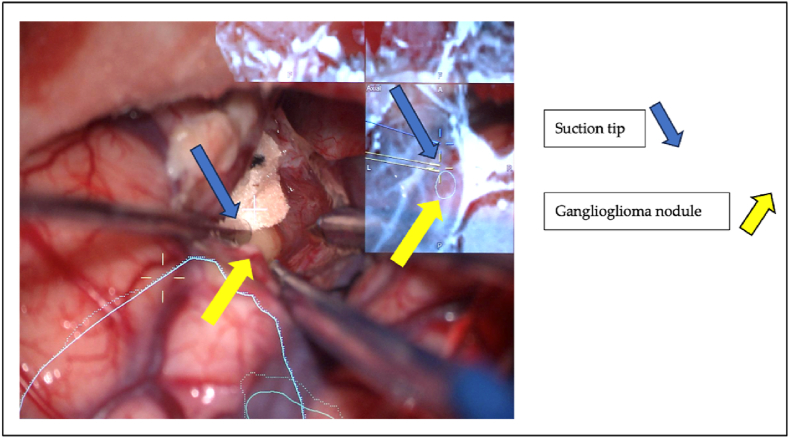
Tailored resection of a right temporo-mesial ganglioglioma: the suction tip is navigated and seen on the 3-plan AR MRI images as a yellow pointer with a yellow cross on the tip. This correlated well with the yellow ganglioglioma seen on the intraoperative fotograph.
